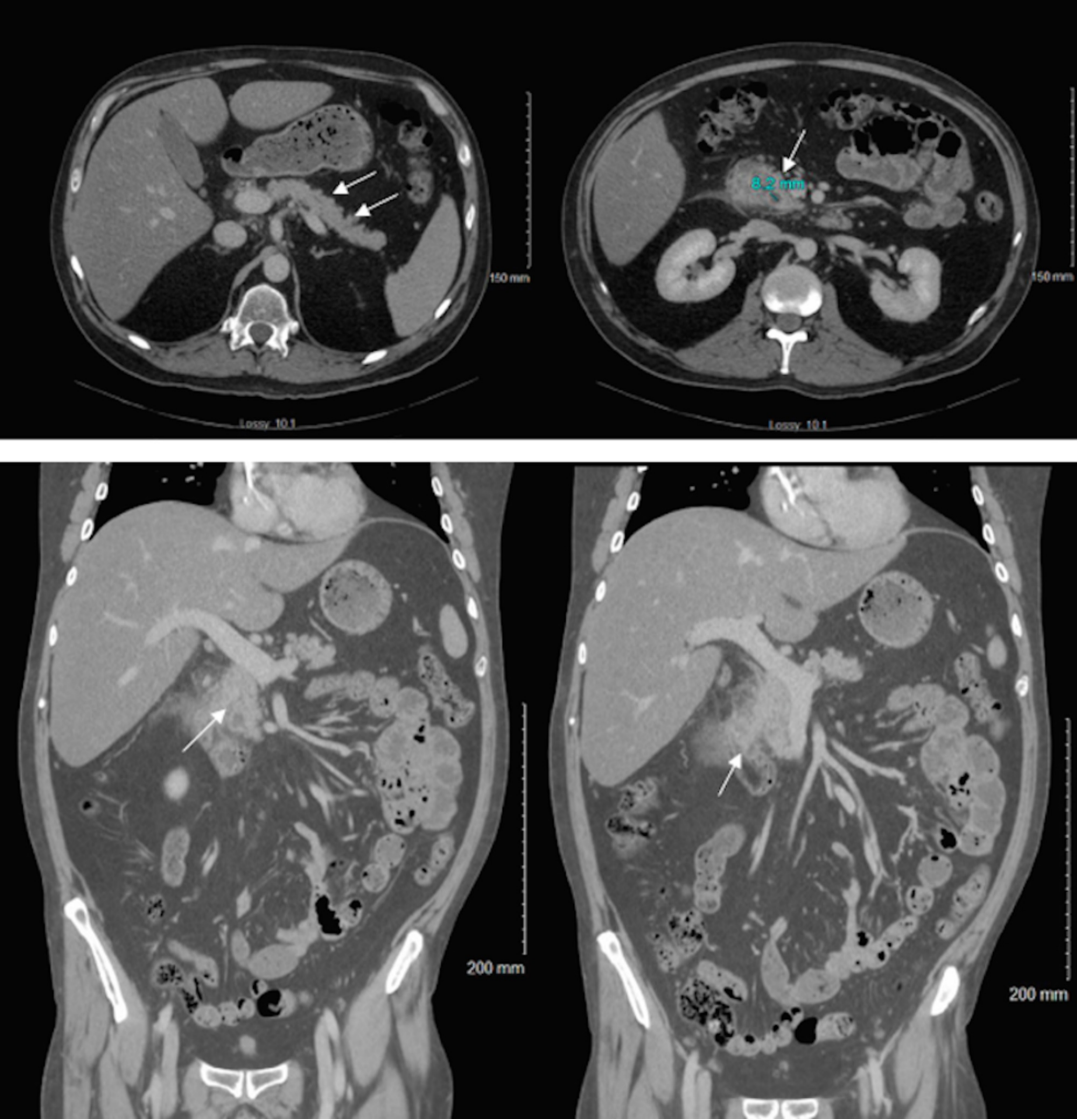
Abdominal computed tomographic (CT) images of acute interstitial pancreatitis Abdominal computed tomography without contrast showing diffuse swelling (arrows, top left) of the pancreas with fat stranding and an 8.2mm fluid collection within the pancreatic head likely pseudocyst (arrow, top right). Coronal sections (arrows, bottom right and left) consistent with acute interstitial pancreatitis but no pancreatic duct stone or ductal dilatation. Duodenal thickening is seen around the head of the pancreas (bottom right).
